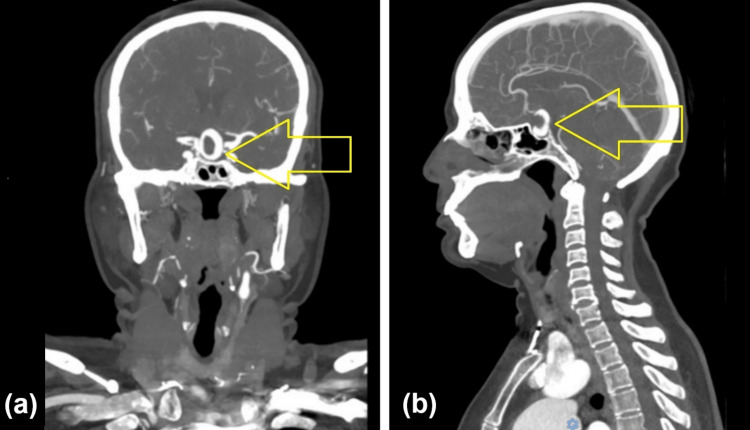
Computed tomography angiography. (A) Coronal image demonstrating a medially directed contrast-filled outpouching from the supraclinoid segment of the right internal carotid artery with a central non-enhancing thrombus (arrow). (B) Sagittal image demonstrating the aneurysmal sac with central thrombosis and suprasellar projection (arrow).
